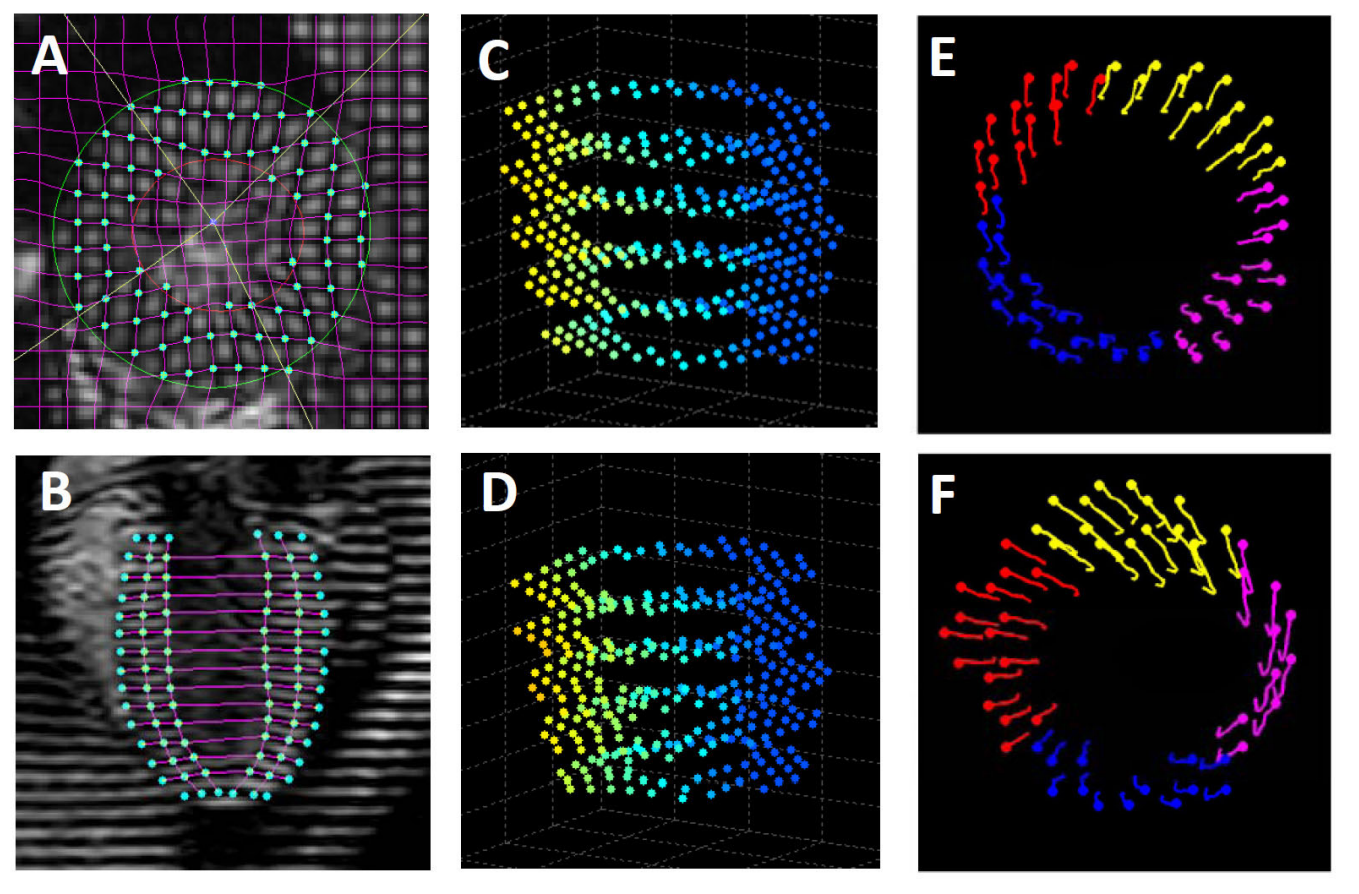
3D MRI tagging image analysis. A&B. Tracking of taglines in tagged short- and long-axis images, respectively; C&D. Reconstructed 3D tag points at end-diastole and end-systole, respectively; E&F. Trajectories of regional myocardial wall motion from end-diastole (dots) to end-systole (tails) at base and apex, respectively.
